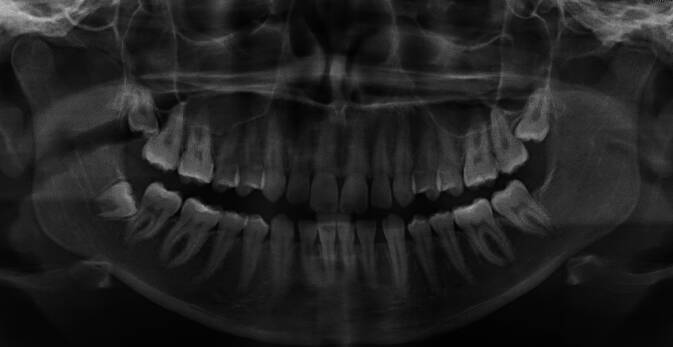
Orthopantomographie: sklerotische Bereiche im linken aufsteigenden Unterkiefer; linker Kondylus mit schweren degenerativen Veränderungen mit Verschmälerung des Gelenkspalts
